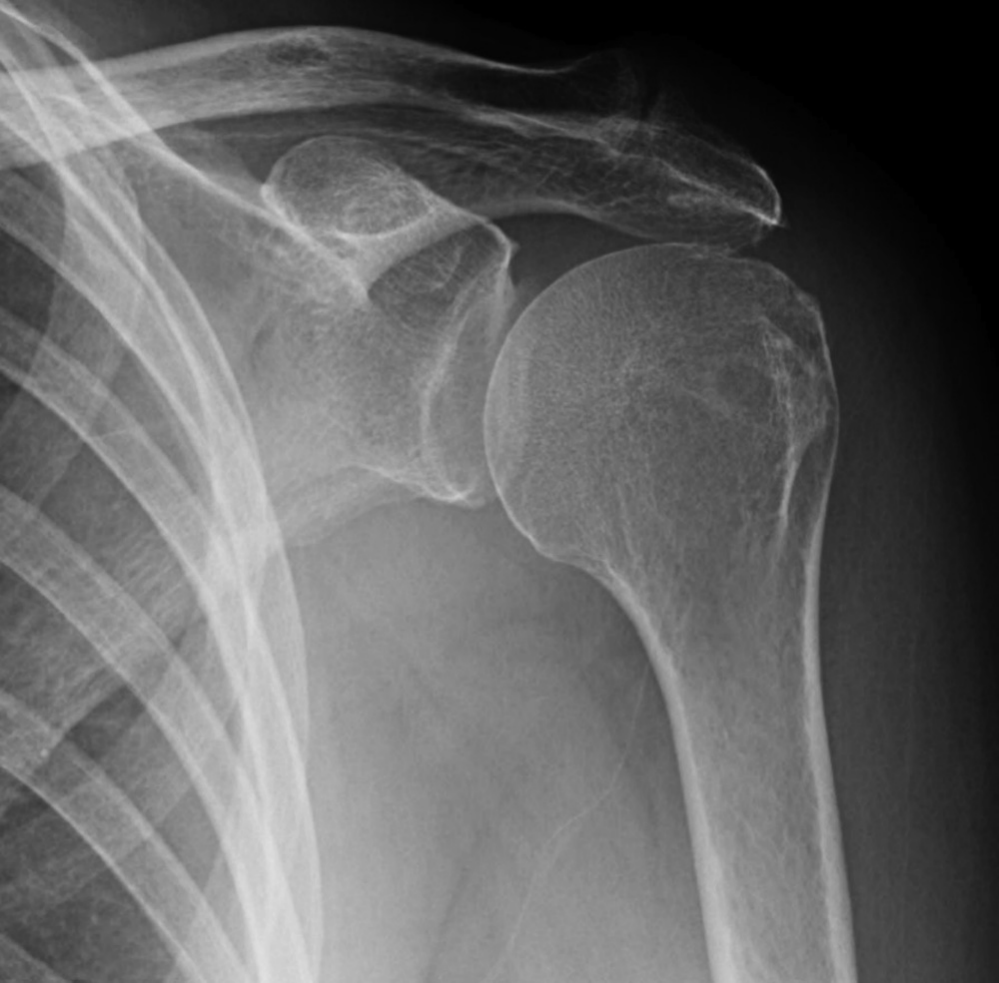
Preoperative anteroposterior radiograph of the shoulder.
